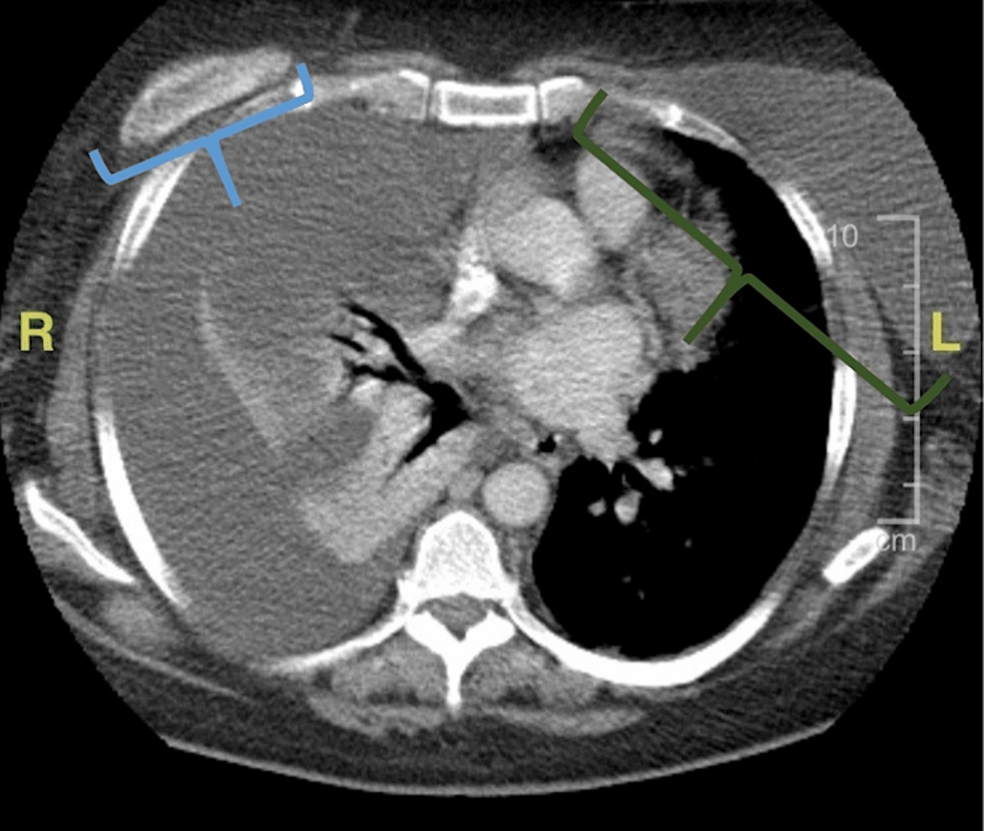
CT Scan of the Chest Computerized tomography scan of the thoracic cavity. The blue bracket on the right encloses the remnant of the silicone breast implant, and the green bracket on the left encloses the intact silicone breast implant. There is a large right pleural effusion causing complete collapse of the right lung and essentially no aeration on the right side.
